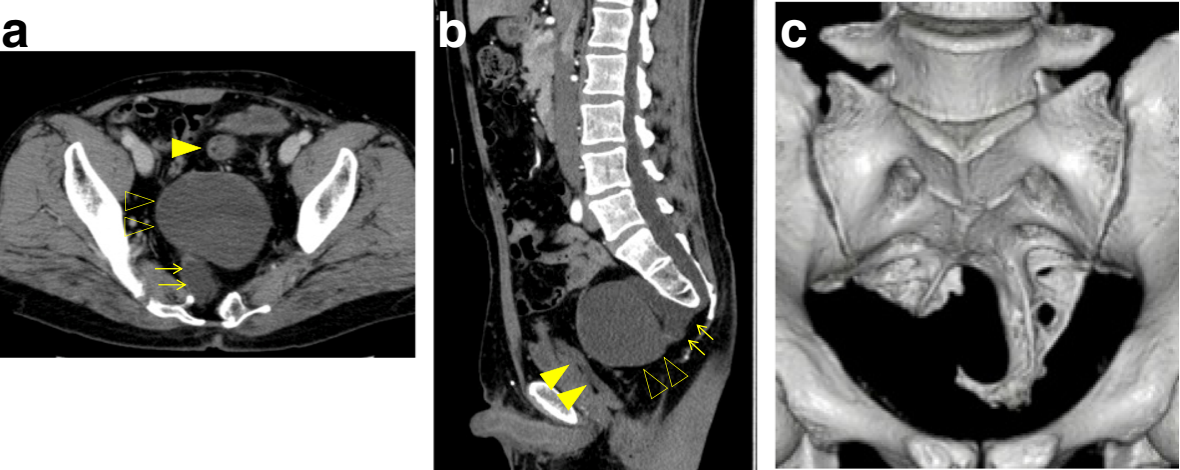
Computed tomography (CT) scan showing a 60 × 30 × 21-mm meningocele (→) which was protuberated anteriorly from the level of S3; a 90 × 85 × 68-mm mass (△) which was located anterior to the meningocele; and a dislocated rectum (▲) (a, b). A partial defect of the sacrum with deformity was detected (c)
